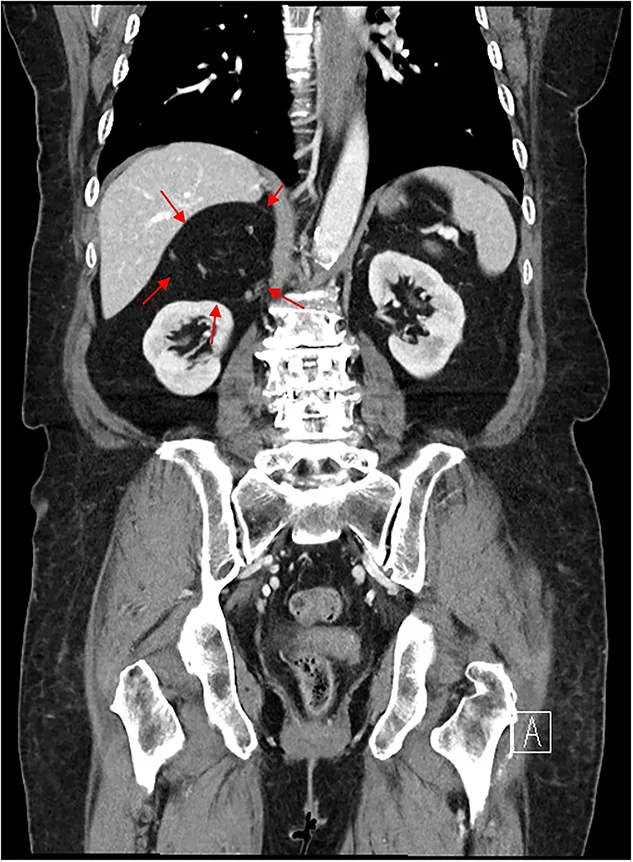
Contrast-enhanced abdominal CT showing a well-defined, fat-containing mass in the right adrenal region (arrows); the lesion demonstrates heterogeneous attenuation with focal soft-tissue components and was initially interpreted as an adrenal myelolipoma.
